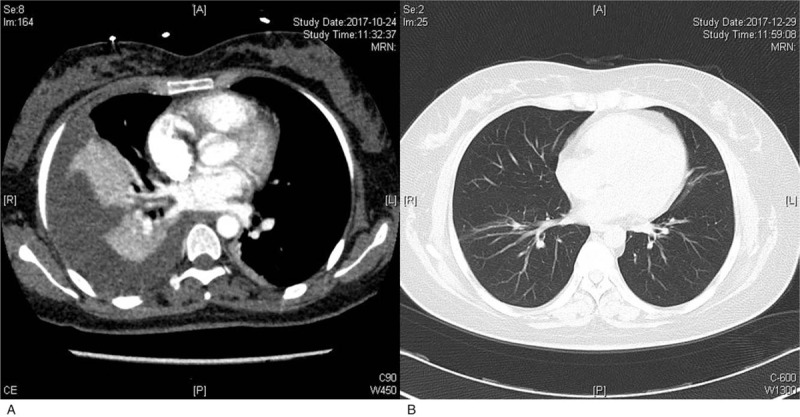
The changes of pulmonary computed tomography images. A: Computed tomography angiography of the aorta showed a large amount of pleural effusion in the right thoracic cavity and partial right pulmonary collapse; B: At the 2-month follow-up visit, her pulmonary computed tomography revealed that the pleural hemorrhage had subsided.
